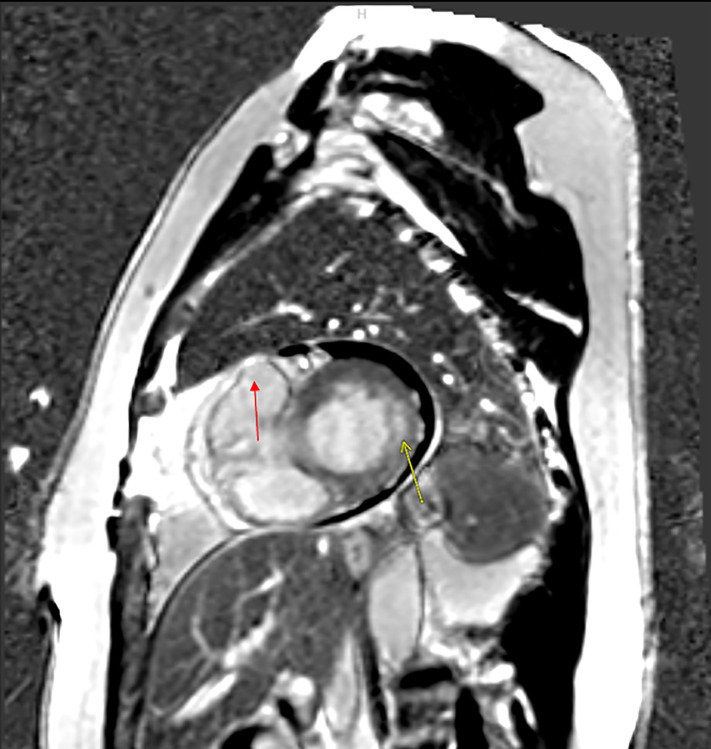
Cardiac Magnetic Resonance Imaging (CMRI) images demonstrating 2
